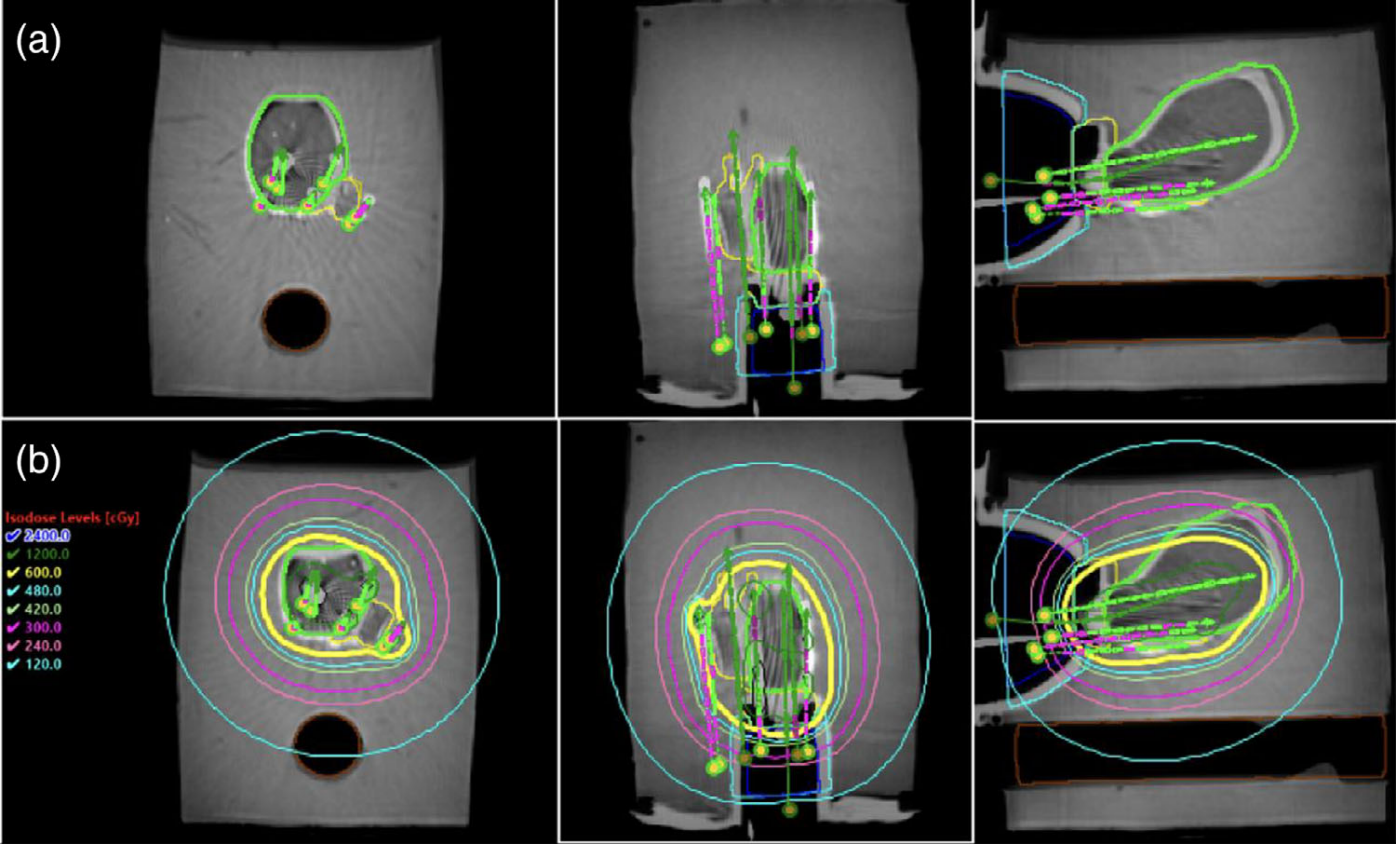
(a) post‐implant CT and T2w‐MR registration displaying uterus (green) and CTV_HR_ (yellow) contours with digitized applicators (green+ purple); (b) dose plan overlay showing isodose levels superimposed on the registered image.
